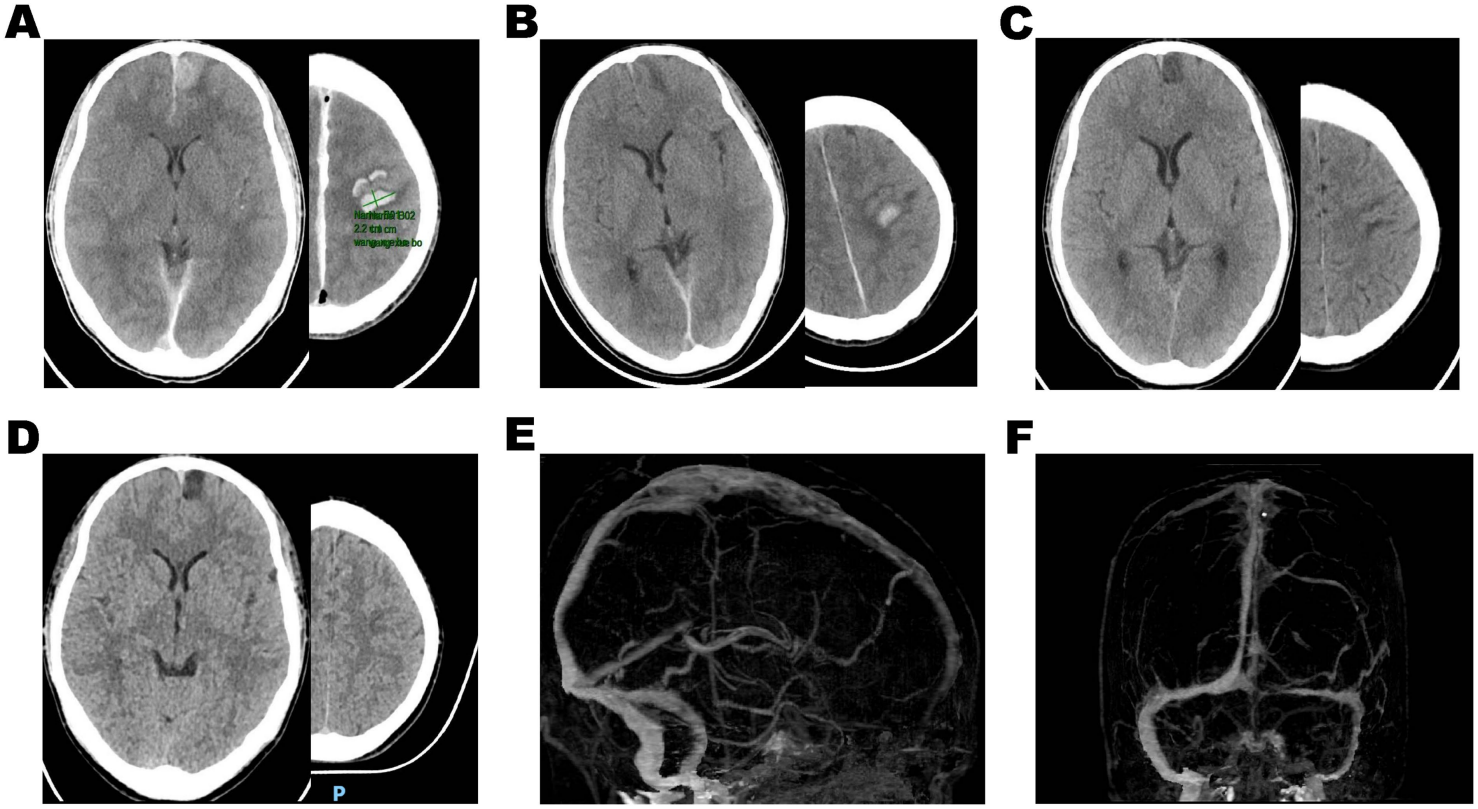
(A) Postoperative head non-contrasted CT demonstrated multiple bleeding in the left frontal lobe, and the volume of hematoma did not enlarge. (B–D) The cerebral hematoma gradually absorbed after continuous urokinase direct thrombolysis in the venous sinus following the thrombectomy. Three months after discharge, cranial CTV showed complete recanalization of the superior sagittal sinus (E) and sinus confluence (F).
